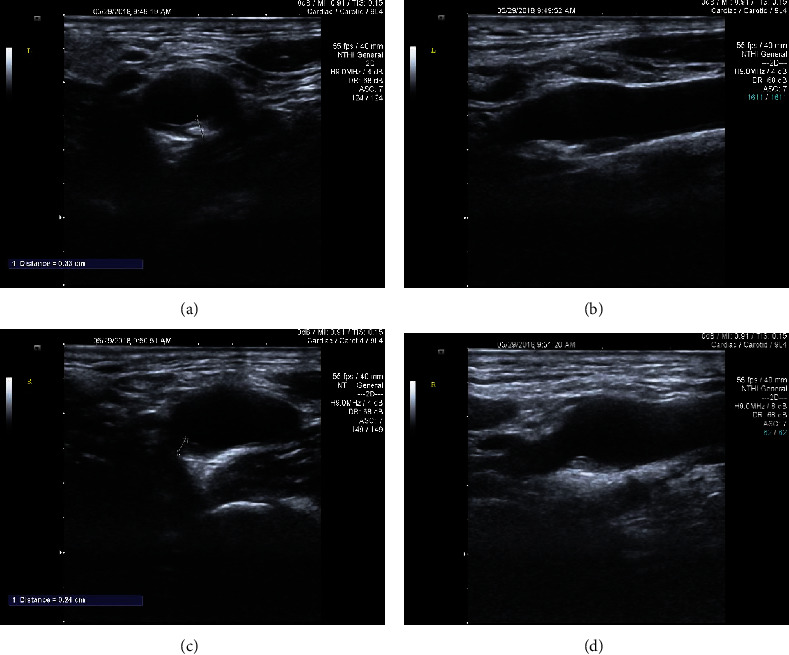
Four different ultrasound images of plaques from a patient. (a) Transverse section of a plaque in the left carotid artery; (b) longitudinal section of a plaque in the left carotid artery; (c) transverse section of a plaque in the right carotid artery; (d) longitudinal section of a plaque in the right carotid artery.
